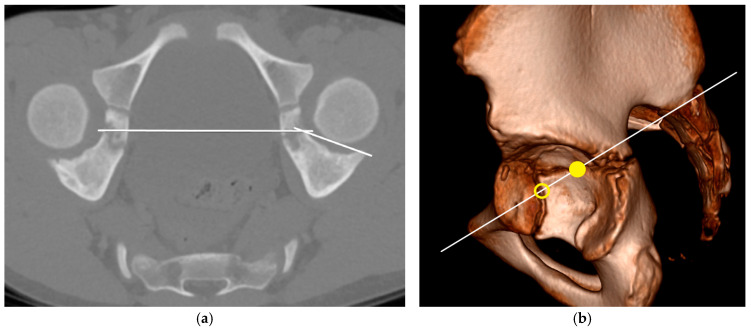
(a) Reformatted CT scan for the measurement of the PSI, (b) Plane of the PSI with intersection of the triradiate cartilage in the posterior (point) and inferior limb (circle).
